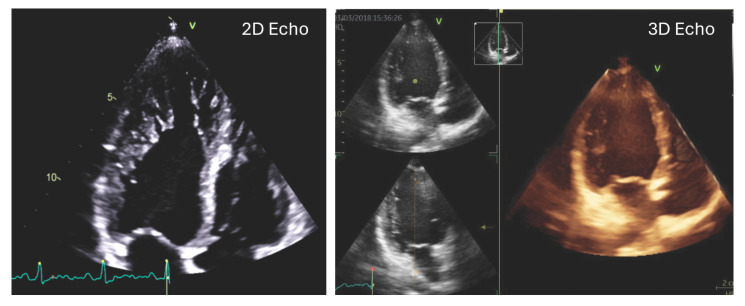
Representative 2D and 3D transthoracic echocardiographic images of a patient with left ventricular hypertrabeculation.
